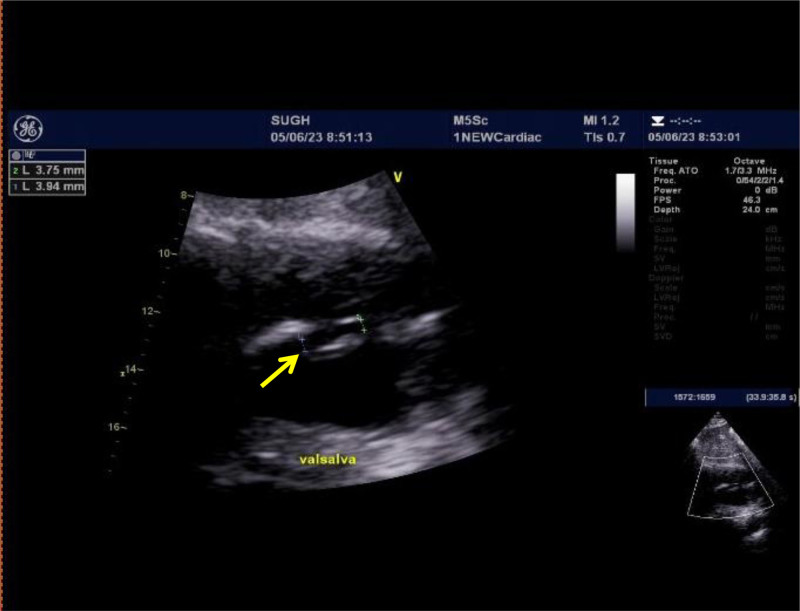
Cardiac ultrasound shows separation of the primary and secondary septum of the atrial septum after the Valsalva maneuver, with about 3 mm in width. So PFO is suspected. PFO = patent foramen ovale.
